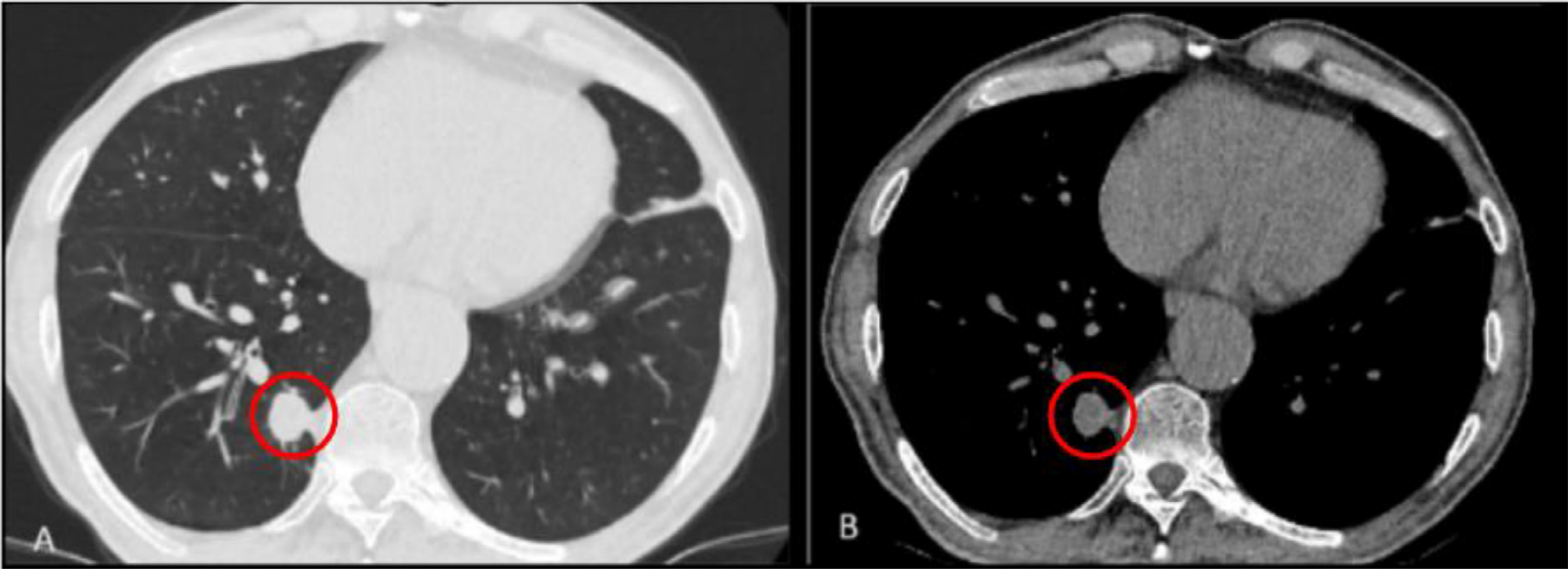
(A) Lung window of the thoracic CT of the first patient 1 month after the cryoablation B) Mediastinal (soft tissue) window of the same thoracic CT. Normally, an early increase in surface area is seen, which peaks at 1 month. During the healing process, the ablation zone is transitioning from a ground-glass appearance to a more defined, nodular pattern (area into the circle).
